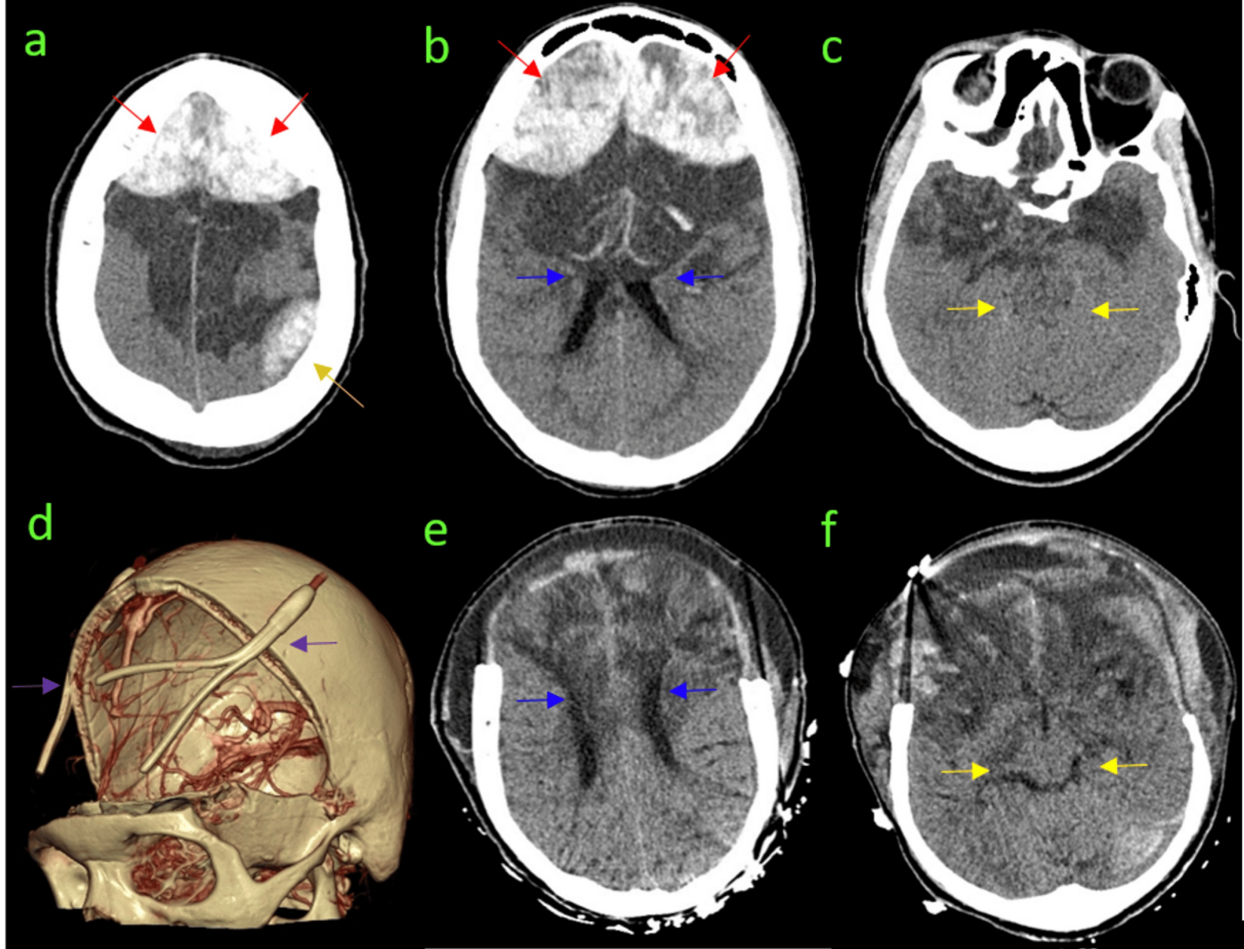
Admission and Post-operative CT (a-c) CT images depict a large bifrontal epidural hematoma (red arrows) and a left posterior parietal mixed-density epidural hematoma (orange arrow). There is a severe mass effect with the complete effacement of bilateral sulci and ventricular compression (blue arrows), indicating increased intracranial pressure. Entrapment and partial effacement of the basal cisterns (yellow arrows) are also noted. (d) A post-operative 3D reconstruction demonstrates the extensive craniotomy with bilateral Jackson-Pratt (JP) drains (purple arrows). (e, f) Post-operative CT axial image showing successful decompression with decreased effacement of skull base cisterns (yellow arrows) and ventricular system (blue arrows).
